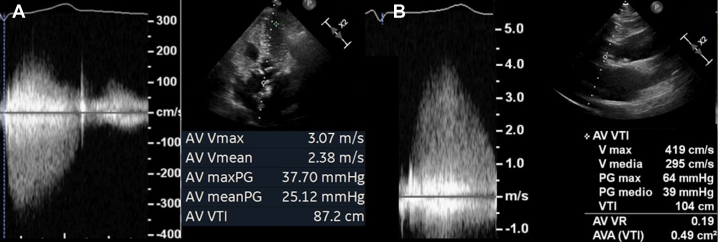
Apparent Low-Flow Low-Gradient Aortic Stenosis The right parasternal approach (B) solved the aortic valve area (AVA)/gradient inconsistencies (A).
